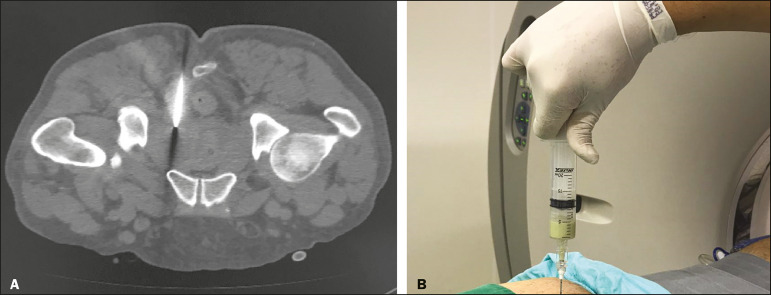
Computed tomography-guided transgluteal access performed with the patient in the prone position (A) and aspiration of purulent content (B).
